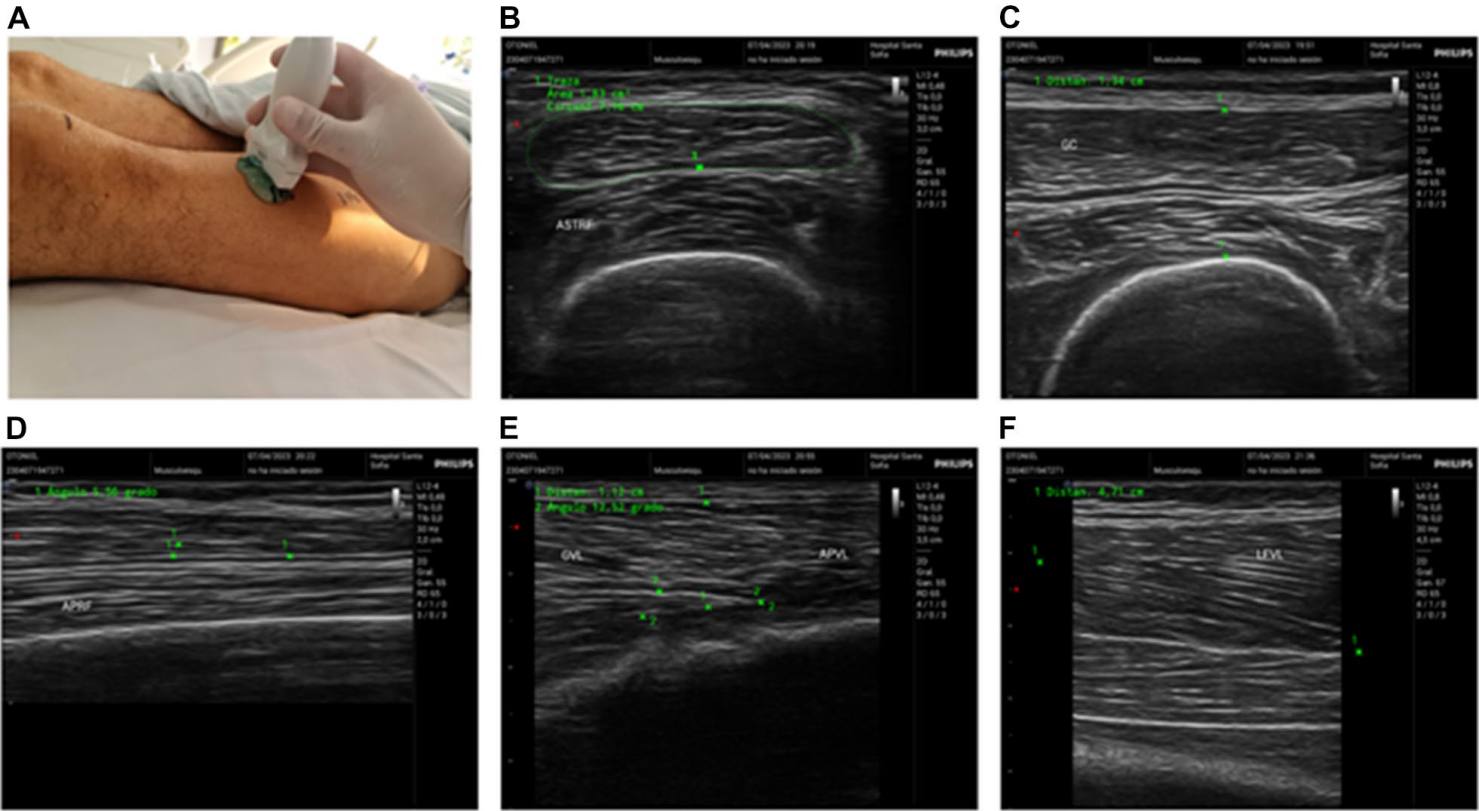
Assessment of quantitative and qualitative measurements of the quadriceps using ultrasound. A Measurement of the midpoint and distal third of the quadriceps, skin marking for sequential measurements, and muscle evaluation in the transverse axis with a high-frequency linear transducer. B Cross-sectional area of the rectus femoris. C Thickness of the rectus femoris and vastus internus. D Pennation angle of the rectus femoris. E Thickness and pennation angle of the vastus lateralis. F Length of the fascicle of the vastus lateralis using a linear extrapolation technique
